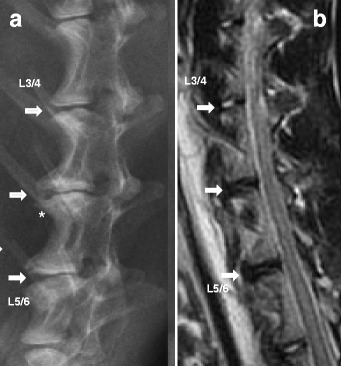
Representative lateral radiographs and magnetic resonance image (MRI) of intervertebral discs at 6 months after surgery. a The experimental segments showed obvious and various degrees of disc space narrowing. Osteophyte formation (*white asterisk*) was evident at the margin of the vertebral bodies. b Significant low T2-weighted signal intensity was clearly visible in the experimental segments after surgery. The *arrows* indicate the operated disc
